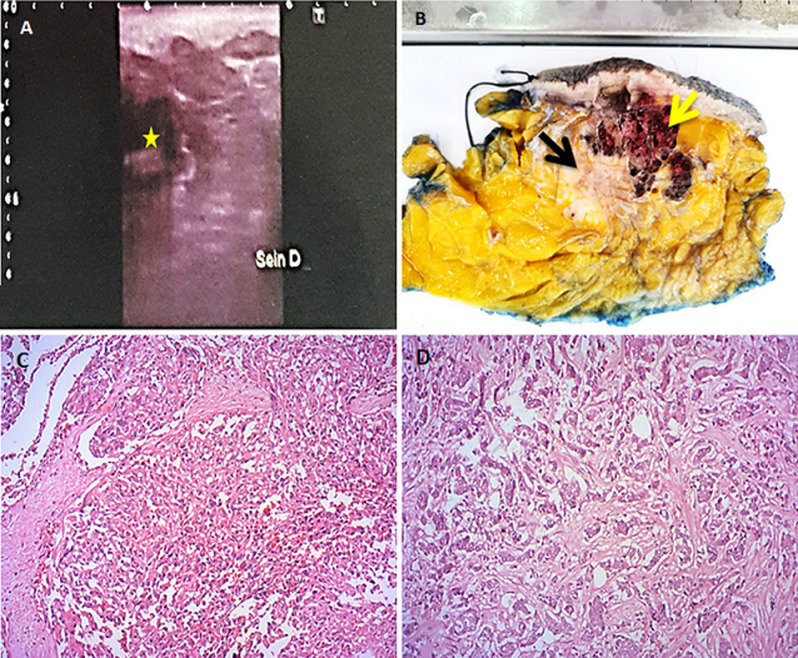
A) ultrasound image of the right breast: nodule with heterogenous echostructure (yellow asterisk); B) gross examination of the two adjacent tumors: spongy and hemorrhagic well-defined tumor (yellow arrow) adjacent to a second grayish-white and irregular tumor (black arrow); C) angiosarcomatous proliferation: anastomosing and irregular vessels with intraluminal tufting (HEx200); D) area of invasive carcinoma of no special type: cords and glandular structures composed of atypical cells (HEx200)
